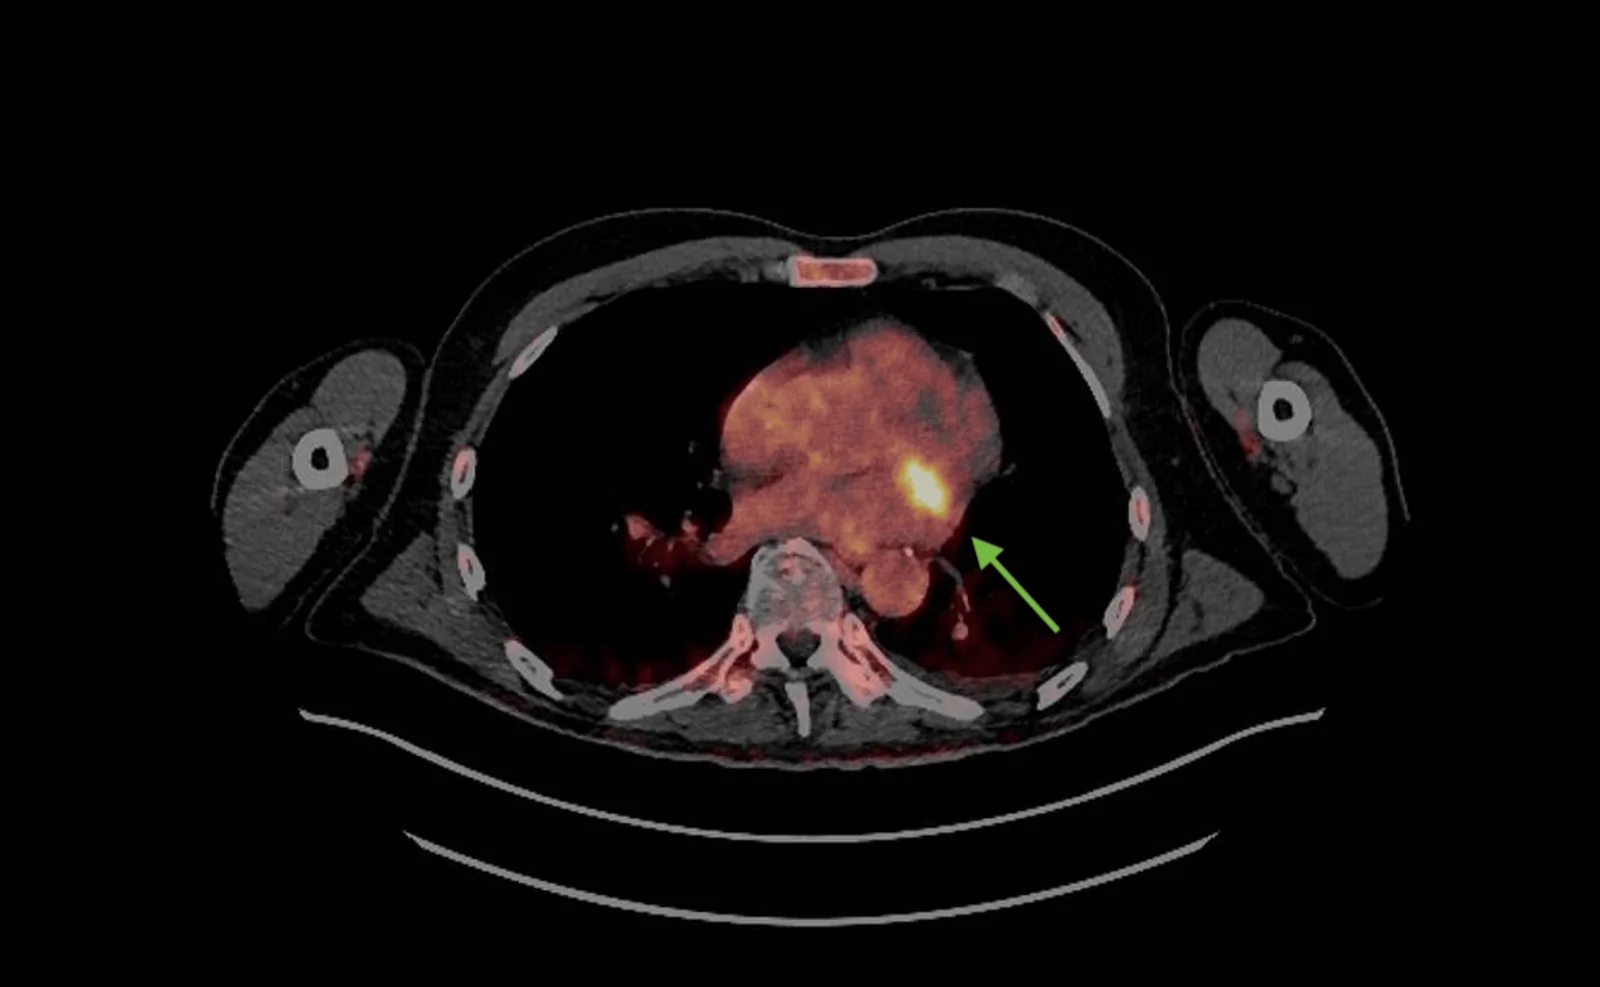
18F-FDG-PET/CT showing a lesion in the left ventricle with high metabolic rate 18F-FDG-PET/CT: fluorodeoxyglucose positron emission tomography/computed tomography
